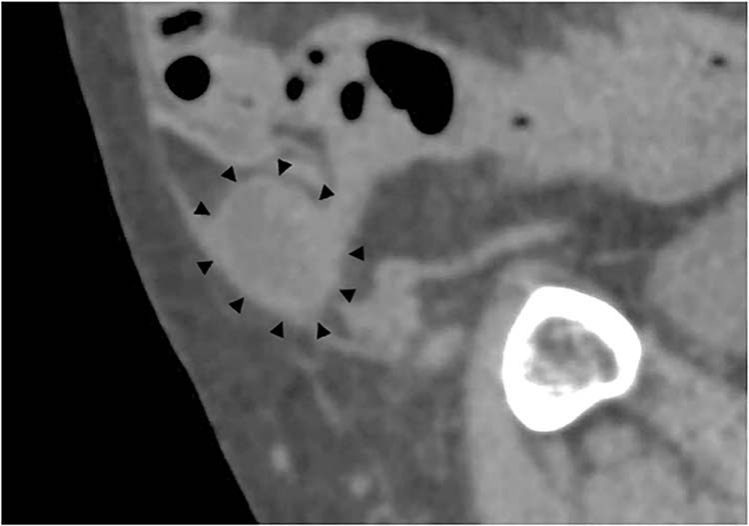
Sagittal section of contrast-enhanced CT; the black triangles indicate the incarcerated small bowel.
